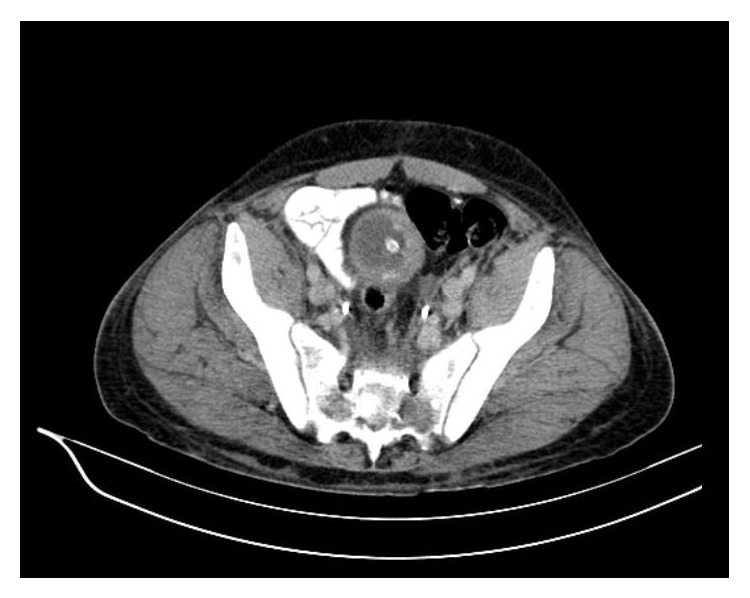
Pelvic CT demonstrating enlarged lymph nodes in the pelvis, more pronounced in the left iliac region.
